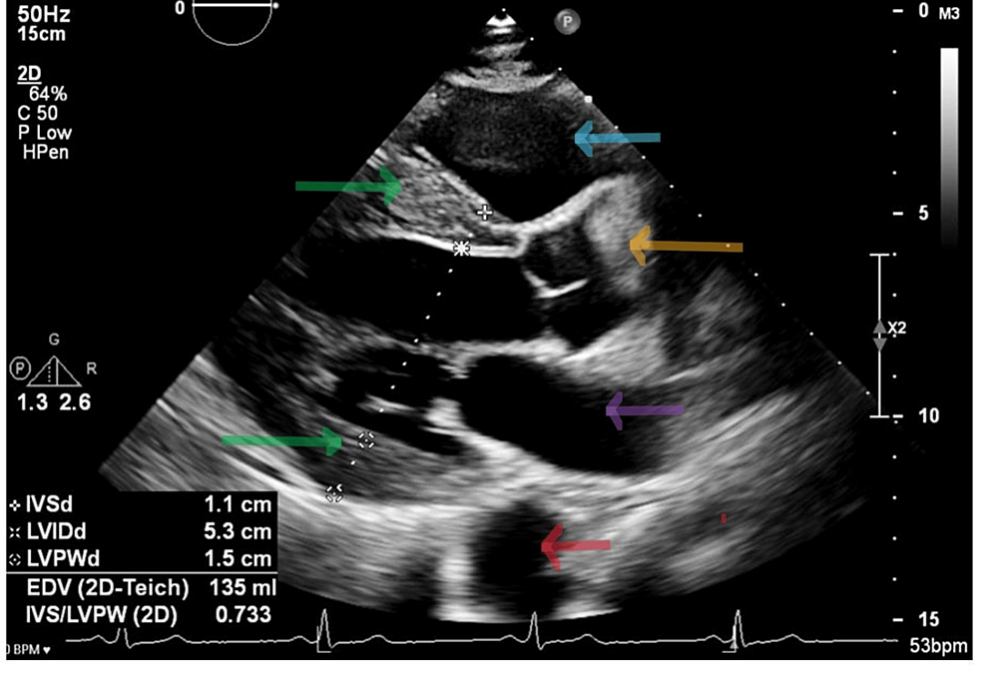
Parasternal long axis. The green arrows show mild concentric left ventricular hypertrophy. The left ventricle is not dilated. The blue arrow shows the left atrium. The orange arrow shows the proximal aorta. The purple arrow shows the left atrium. The red arrow shows the descending aorta.
